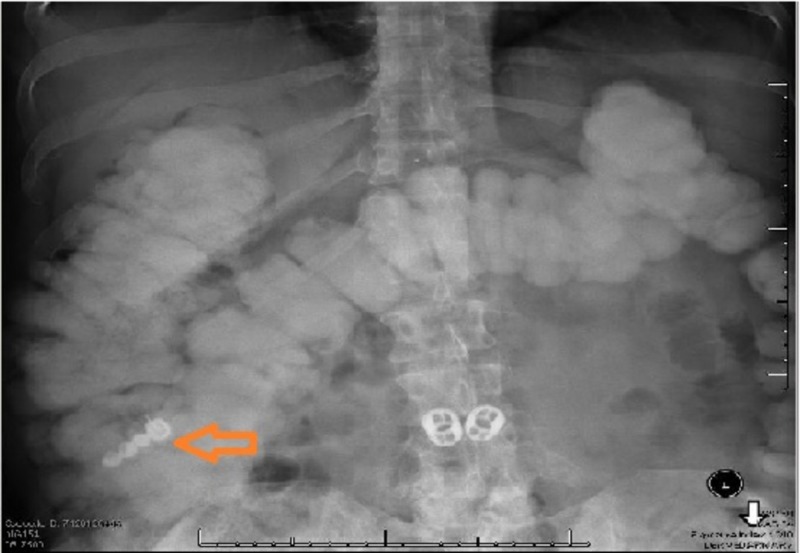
Abdominal X-ray showing the foreign body overlying the right abdomen; it could be within the proximal transverse colon, or could be within the right colon
